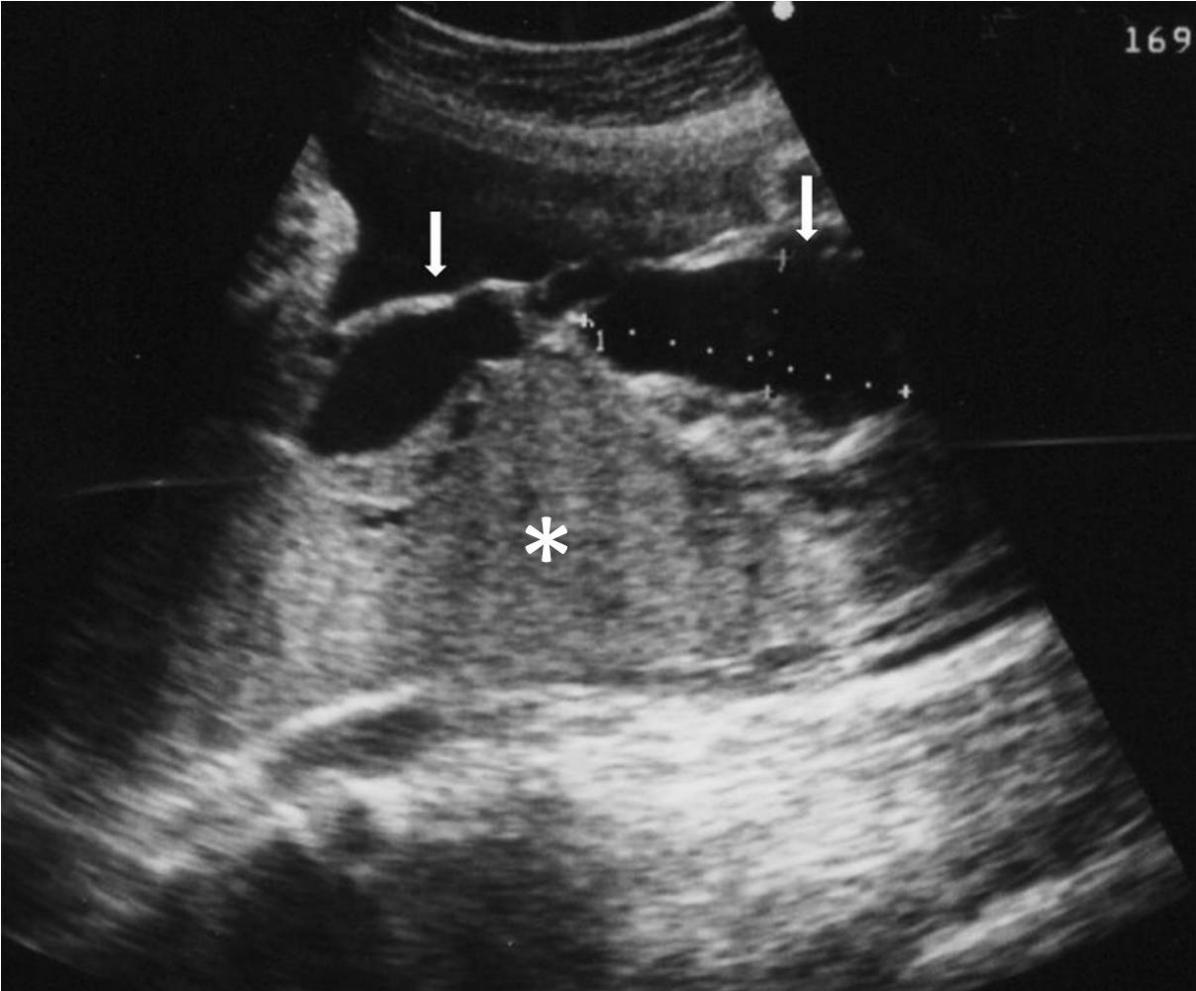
Transabdominal ultrasound study. Placental parenchyma (asterisk) and dilated subchorionic vessels (arrows) at 35 weeks' gestation. These vessels are up to 2.5 centimeters in diameter.
